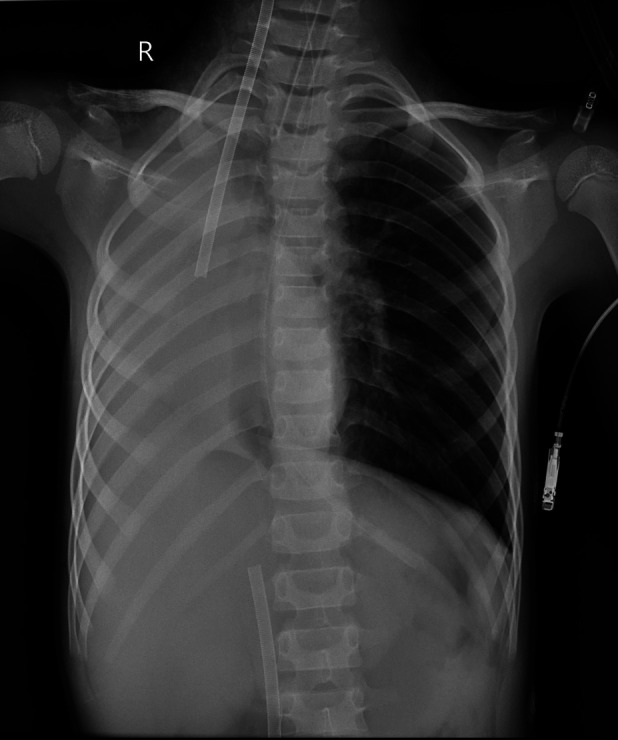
Chest x-ray performed immediately after ECMO initiation.
